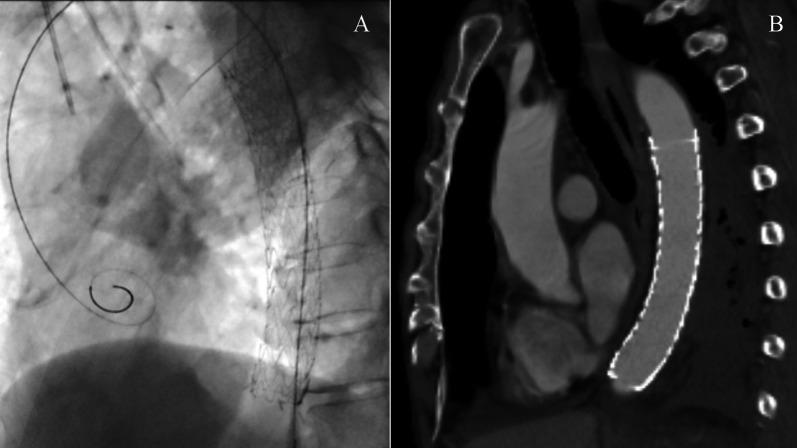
Chest X-ray and contrast-enhanced CT of the stent graft. A Chest X-ray showing the stent graft delivered to the descending aorta. B Five days after surgery, chest contrast-enhanced CT revealed no endoleak or stent migration. CT: computed tomography
